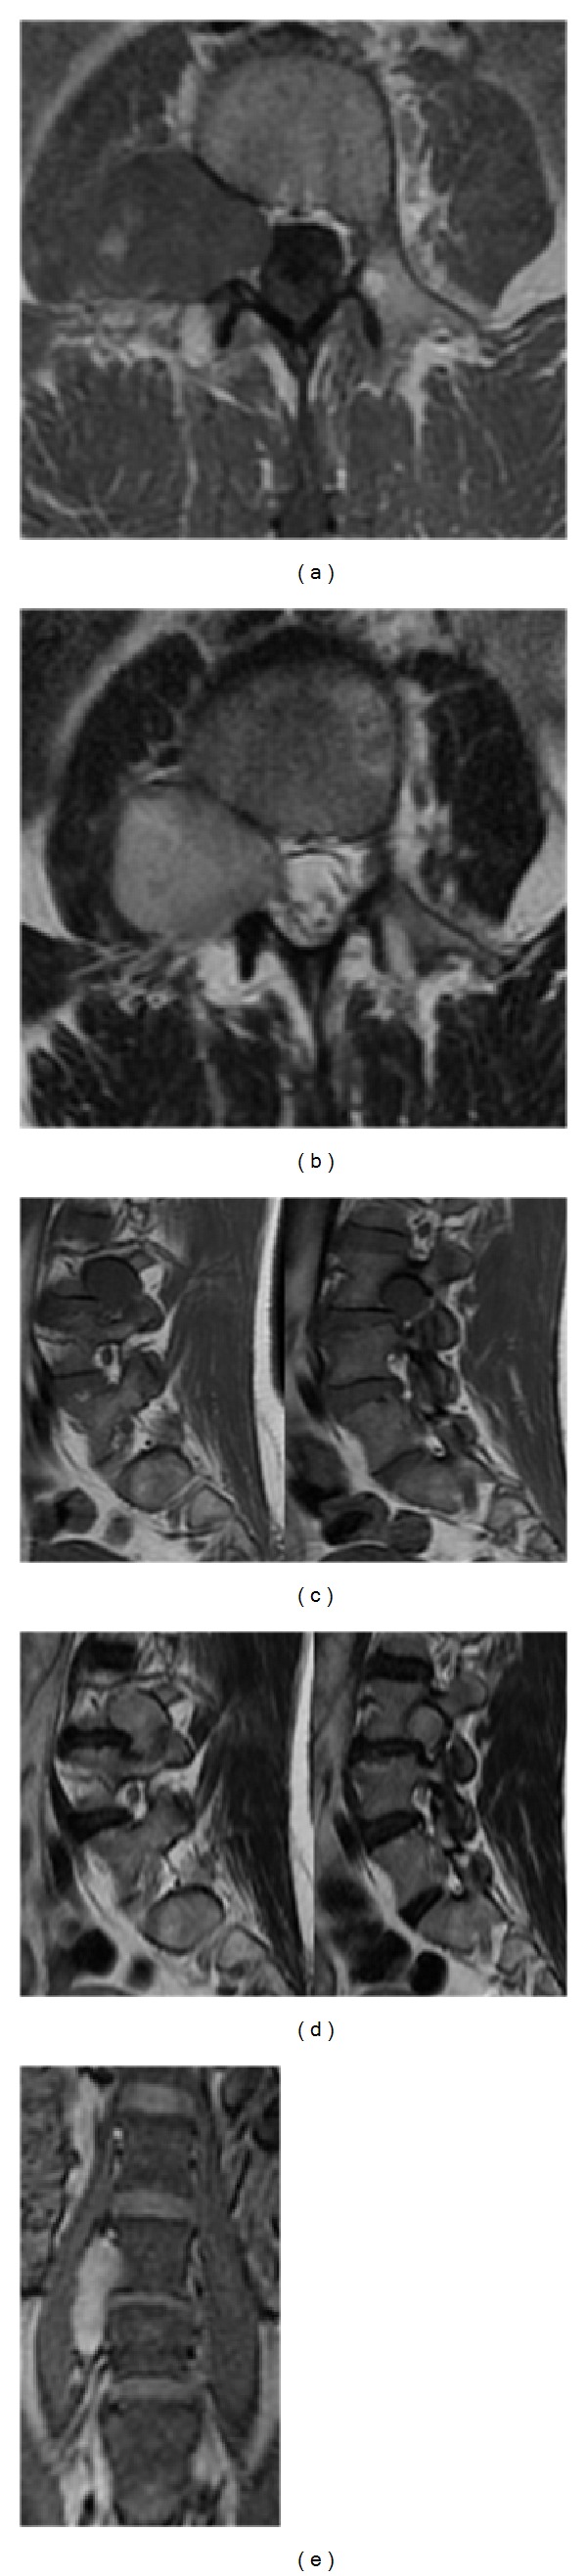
Preoperative MRI documents a right L3/L4 completely extradural dumbbell-shaped tumor with extraforaminal extension into the right psoas muscle: (a) T1-weighted; (b) axial T2-weighted with gadolinium enhancement; (c) sagittal T1-weighted showing extraforaminal and foraminal tumor components; (d) sagittal T2-weighted with gadolinium enhancement showing extraforaminal and foraminal tumor components; (e) coronal view with gadolinium.
